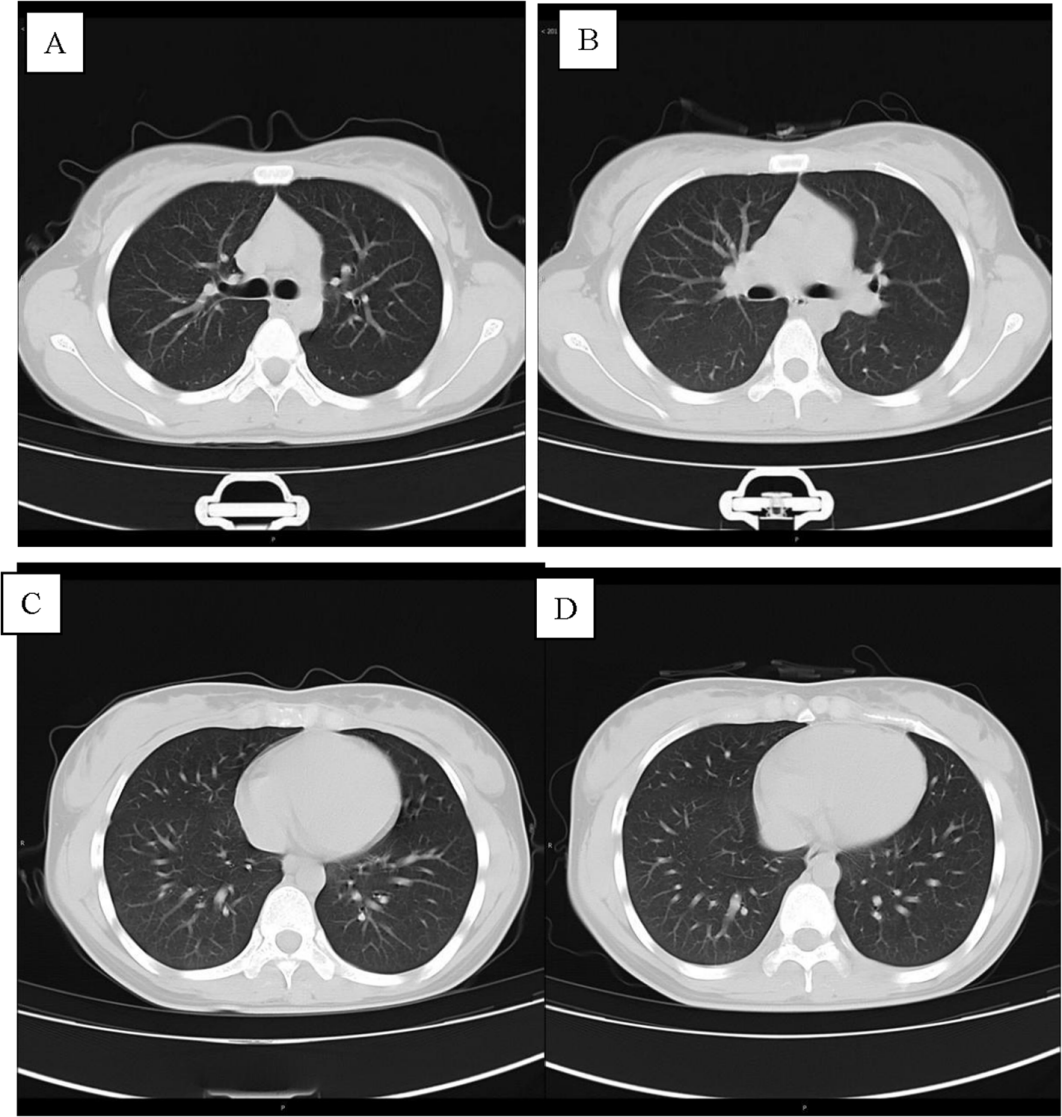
Chest CT images of A 18-year-old girl with COVID-19 infection. a, c Transverse chest CT images showed no abnormalities in both lung on admission. b, d Transverse chest CT images showed no abnormalities in both lungs on discharge
